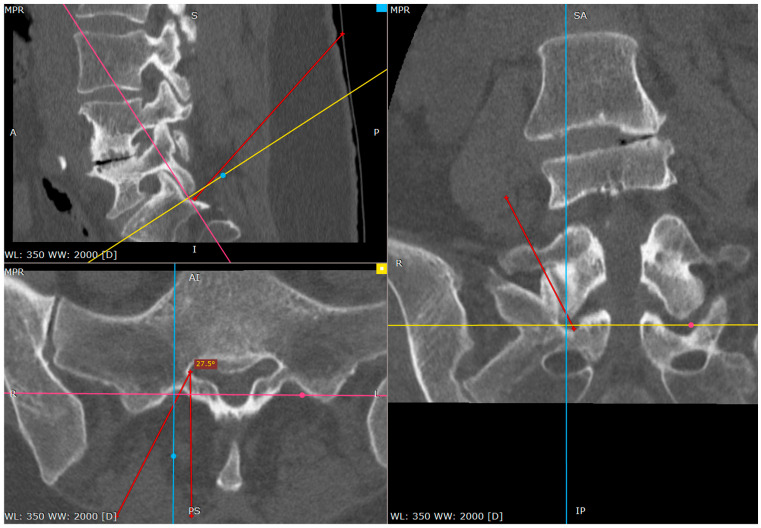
Approach angle and needle trajectory to facet with intent to approach S1 root (CT tri-plane reconstruction).
